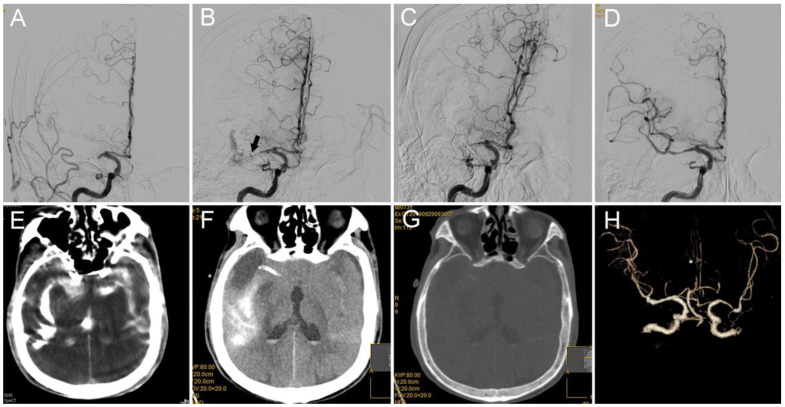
(A) DSA shows occlusion of the M1 segment of the right MCA. (B) A microcatheter is advanced into the M1/2 branch (the arrow indicates the tip of the microcatheter), where active contrast extravasation pointing to the location of vessel perforation can be observed (indicated with arrow). (C) After the injection of 400 U thrombin via the microcatheter and no following contrast extravasation, the contrast extravasation is stopped. (D) mTICI 3 reperfusion was achieved after the detachment of the stent retriever and administration of intravenous tirofiban. (E) Severe hyperdensity within the Sylvian fissure and ventricle was observed on C-arm CT performed during the procedure. (F,G) The hyperdense area was dismissed on follow-up CT and disappeared on dual-energy CT, which indicates that it was a contrast agent but not a blood product. (H) Patency of the culprit artery was observed on the follow-up CTA. MCA: middle cerebral artery; mTICI: modified thrombolysis in cerebral infarction; CTA: computed tomography angiography.
